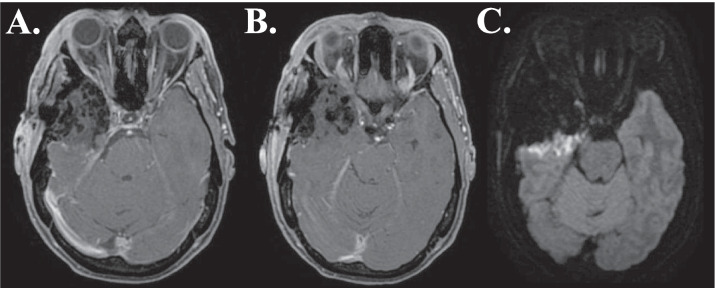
AB: Postoperative (< 48 h) MRI scan (T1W sequence) of patient with medial sphenoid wing meningioma, showing postoperative changes and Simpson grade I resection. C: Postoperative (< 48 h) MRI scan (DWI sequence) of patient with medial sphenoid wing meningioma, showing mild diffusion restriction at the pia-meningioma interface
